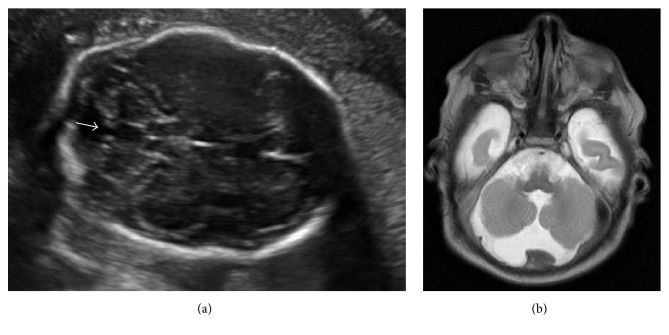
(a) Prenatal axial ultrasound shows the skull shape aberrancy and the presence of vermian agenesis with communication between the 4th ventricle and the cisterna magna (depicted by arrow). (b) Postnatal MRI was significant for hypogenesis of the inferior cerebellar vermis with mild fourth ventricular enlargement which communicates with a retrocerebellar CSF collection.
